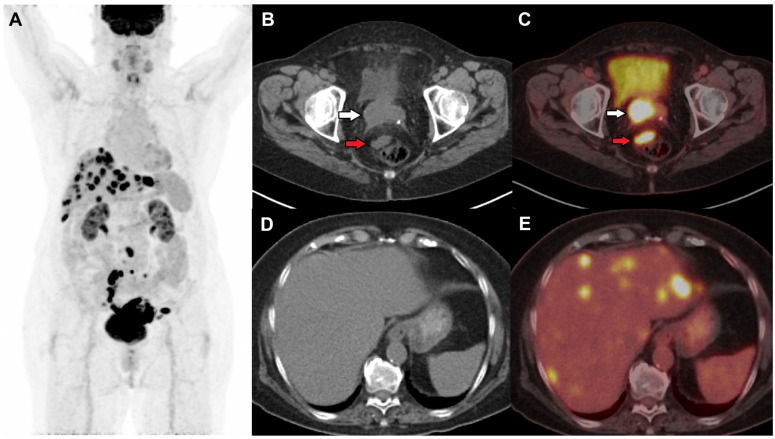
(A) Restaging FDG PET/CT was performed in a female with initial stage 1B2 status post-hysterectomy and bilateral salpingo-oopherectomy and suspected recurrence on local examination and vaginal bleeding 18 months after the surgery. Axial CT and fused PET/CT images show an FDG-avid soft tissue nodule in the right vaginal cuff (white arrow) suspicious of local recurrence and an FDG-avid perirectal node (red arrow) (B,C), and multiple FDG-avid hepatic metastases (D,E).
